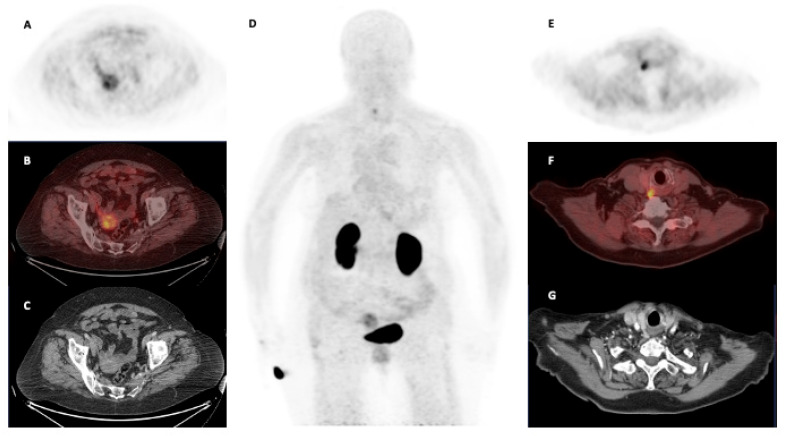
Normal biodistribution of [^68^Ga]Ga-Trivehexin PET/CT in a patient with primary hyperparathyroidism performed at the Department of Nuclear Medicine, Hacettepe University, Ankara, Turkey. PET/CT was acquired 60 min p.i. of 160 MBq of [^68^Ga]Ga-Trivehexin and characterized by low soft tissue uptake ((A,E): emissive trans-axial PET images; (B,F): trans-axial fused PET/CT images; (C,G): trans-axial CT images; (D): maximum intensity projection—MIP). Radiotracer uptake was seen in the kidneys and bladder due to renal excretion. Incidental uptake in a uterine fibroid was also noted (A–C). A focal radiotracer uptake in a parathyroid adenoma was detected.
